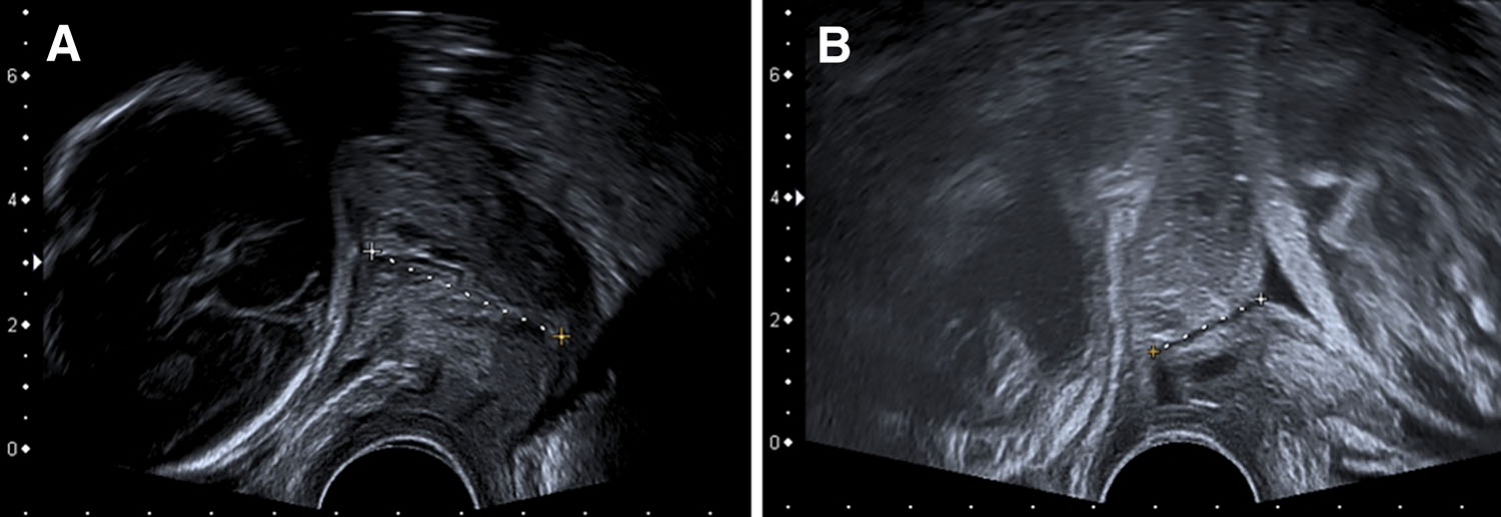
Sonographic cervical length measurement
